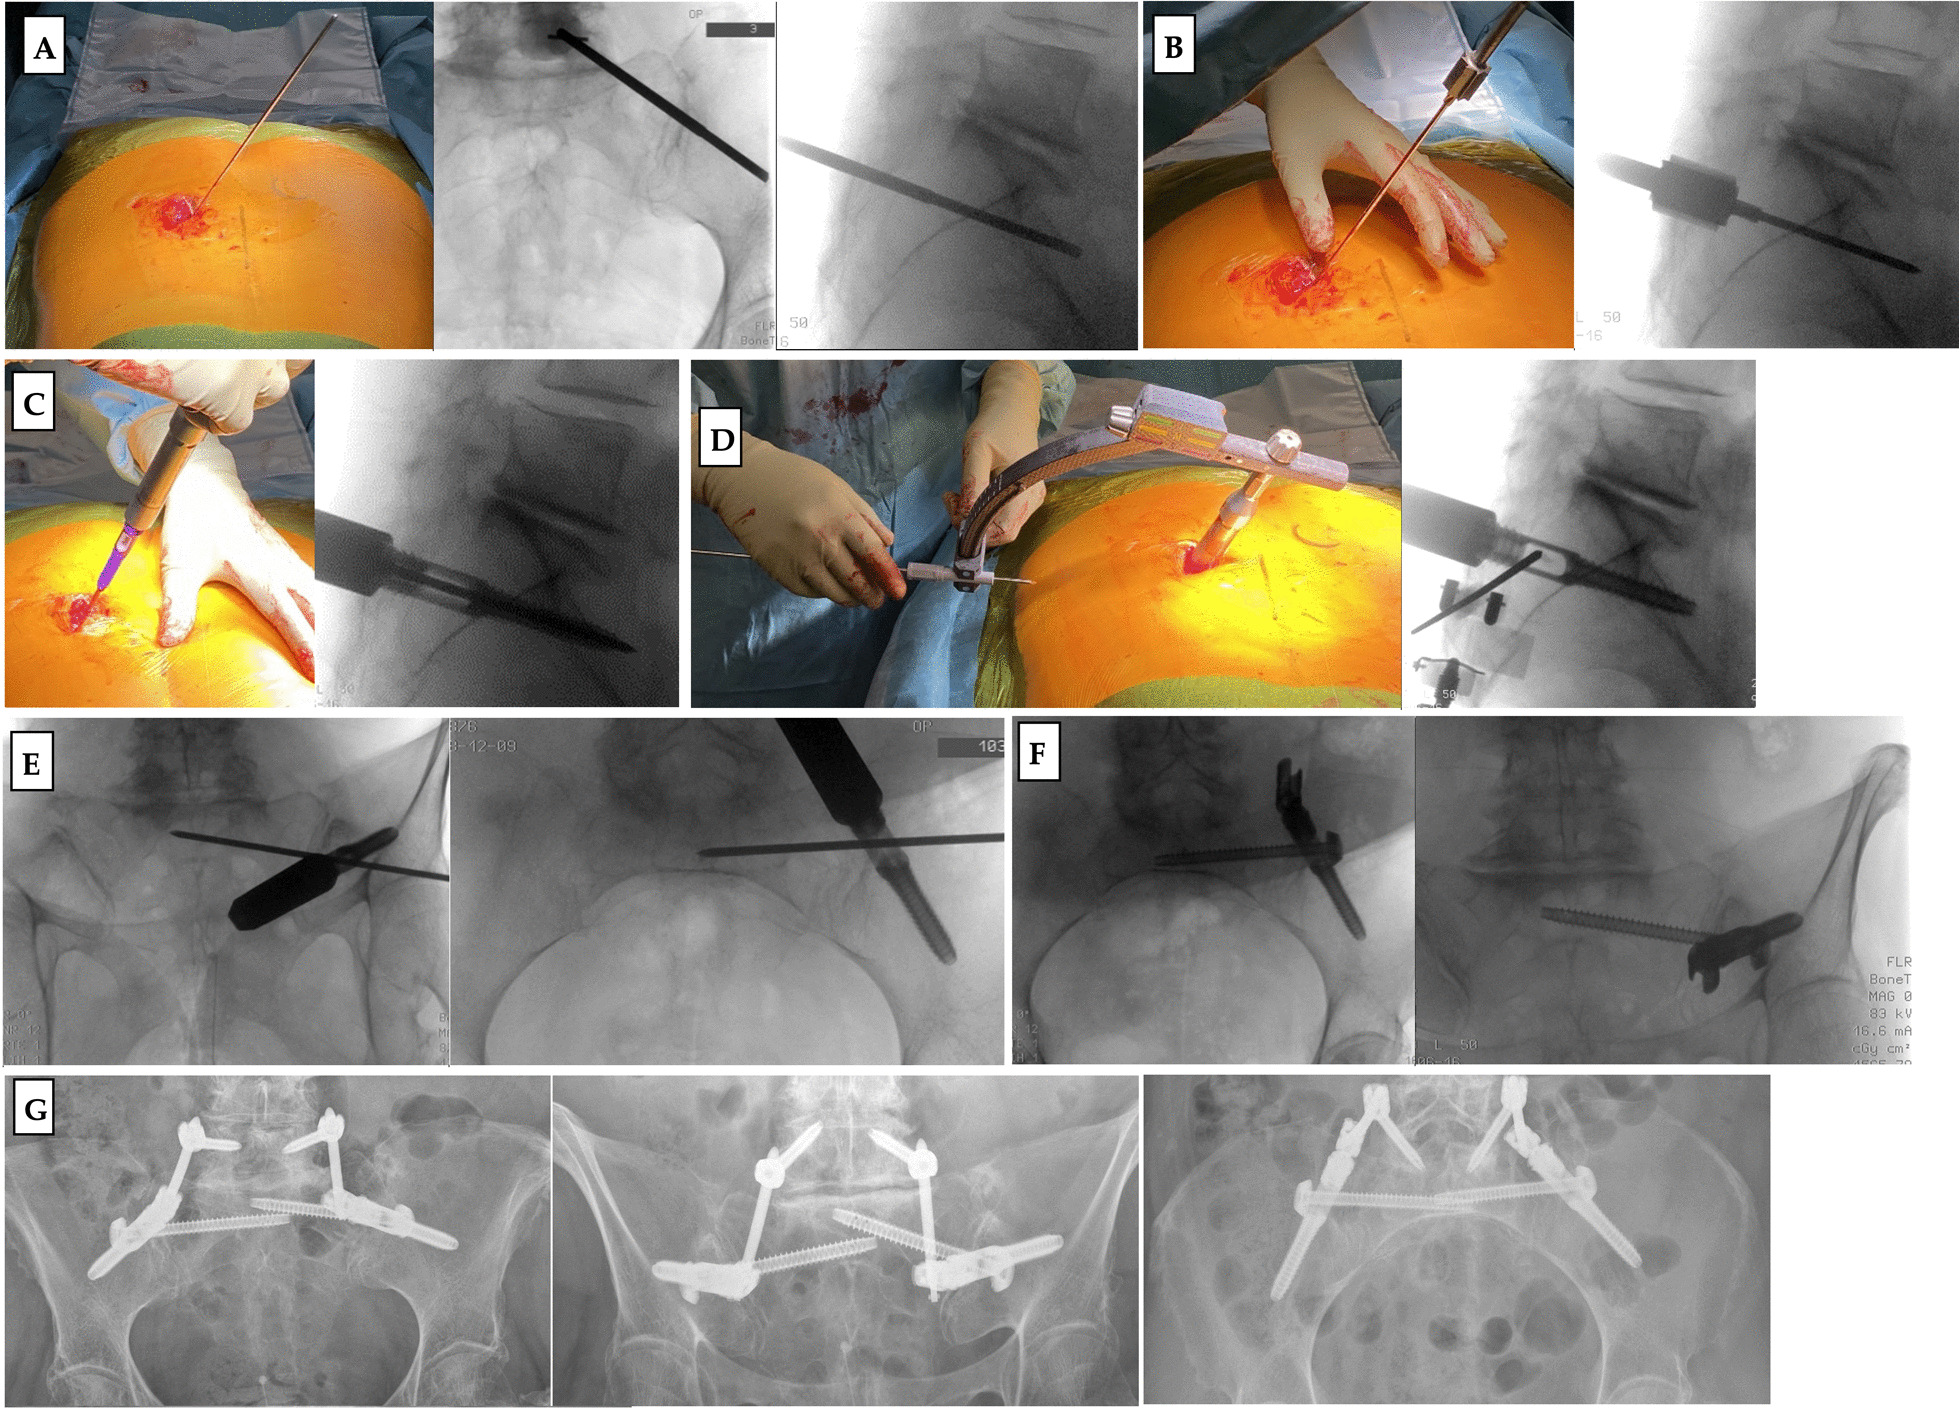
Pictures showing the operative case of 72-year-old female patient with a U-shaped sacrum fracture after a fall from standing hight. a Patient in prone position with the Kirschner wire for the ilium screw in position, x-rays showing correct placement. b Preparation of the entry point of the ilium screw with size determination in lateral x-ray projection. c Insertion of the ilium screw and control of the correct insertion depth with the image intensifier. d Attachment of the guiding device and placement of the Kirschner wire for the iliosacral screw under x-ray control. e Checking final Kirschner wire position in two planes using the image intensifier. f X-ray control after iliosacral screw insertion and adaption of the polyaxial head onto the ilium screw for lumbo-pelvic stabilization. g Postoperative x-ray control after bilateral triangular fixation and lumbo-pelvic stabilization
